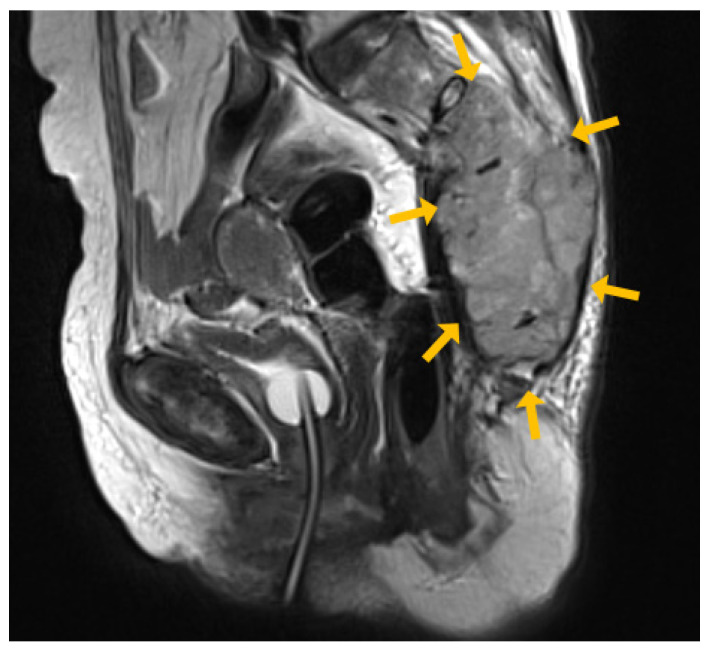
Pelvic MRI. Irregular bone marrow-infiltrated mass in the sacrum.
